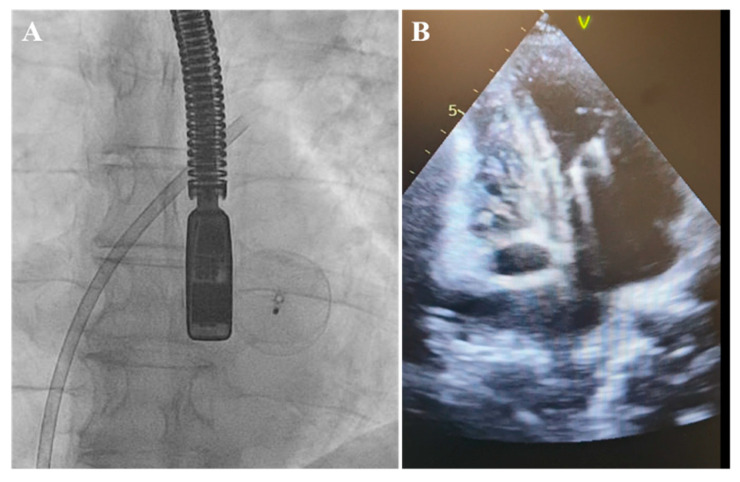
Detached occluder in the projection of the left ventricle. (A) Fluoroscopy. (B) Transthoracic echocardiography.
